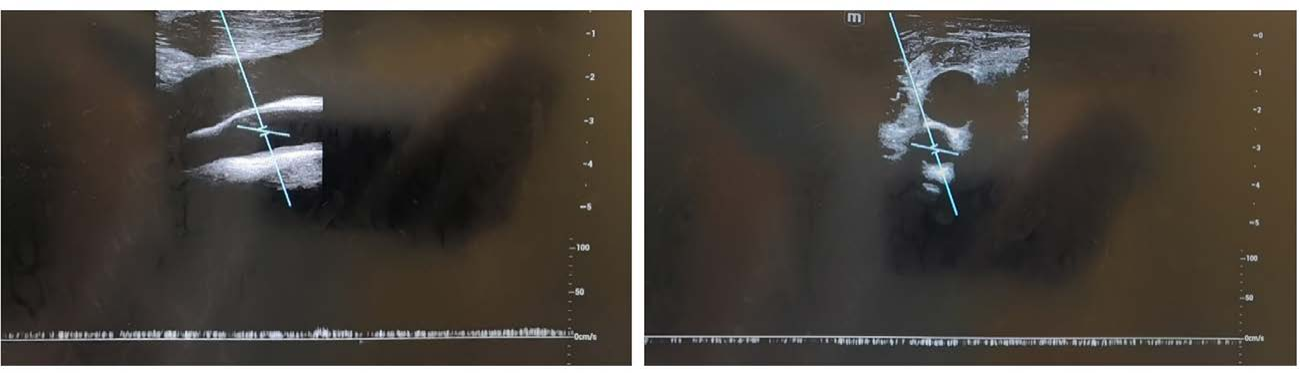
Bedside ultrasound revealed unidirectional pulseless flow in the right common carotid artery.
